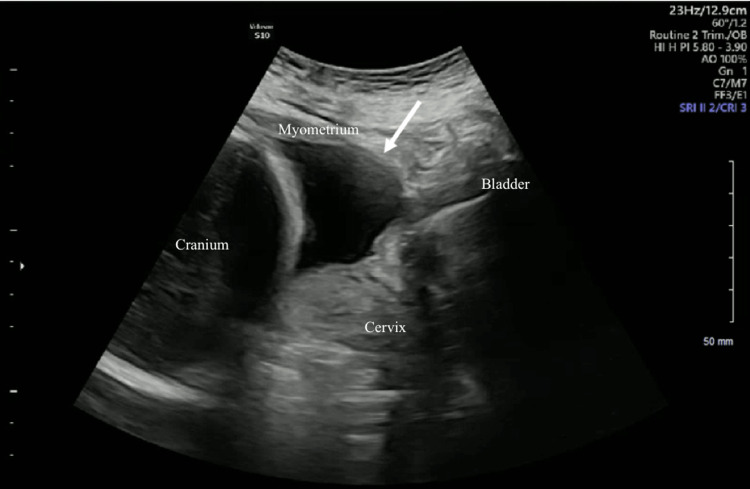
Transabdominal ultrasound of the lower uterine segment. Transabdominal ultrasound of the lower uterine segment showing the myometrium anteriorly abruptly thinning with only a serosal layer remaining with protrusion of the amniotic sac through this defect in the sagittal view.
